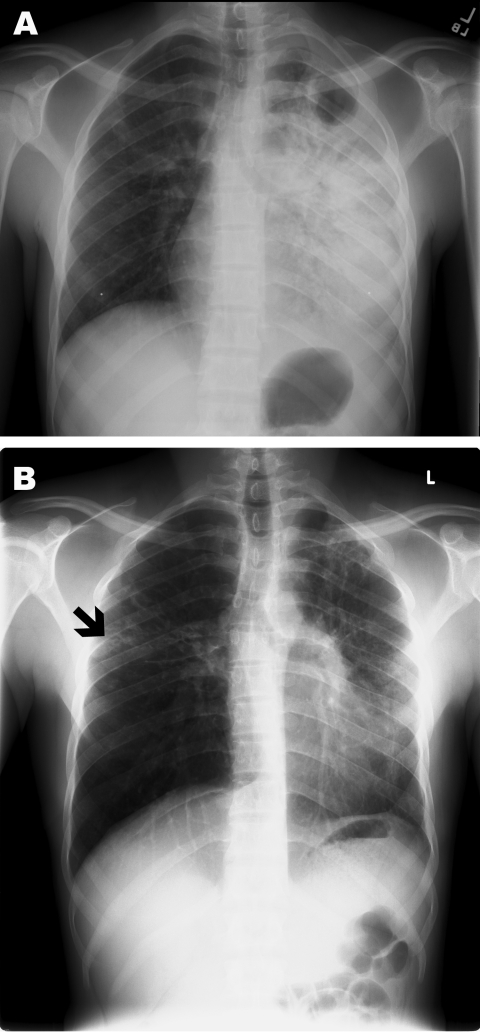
Poster-anterior chest radiographs of patient with multidrug-resistant tuberculosis. A) Radiograph taken at diagnosis, demonstrating dense consolidation of the left lower lobe and lingula. A left apical cavity is present. Minimal change is also noted in the right mid-lung zone. B) Radiograph taken after 5 months of directly observed therapy. Marked clearance is noted on the left; however, a new small cavitary lesion with surrounding infiltrate is noted in the right mid-lung zone (black arrow).
